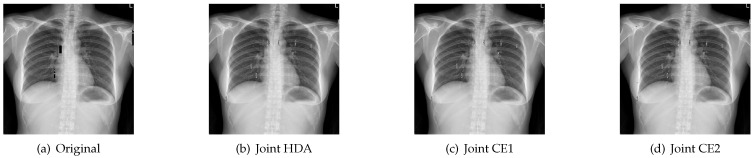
Reconstructed images with different stopping criterions for “X-ray” when $E_{b}/N_{0}$ = 1.5 dB.
